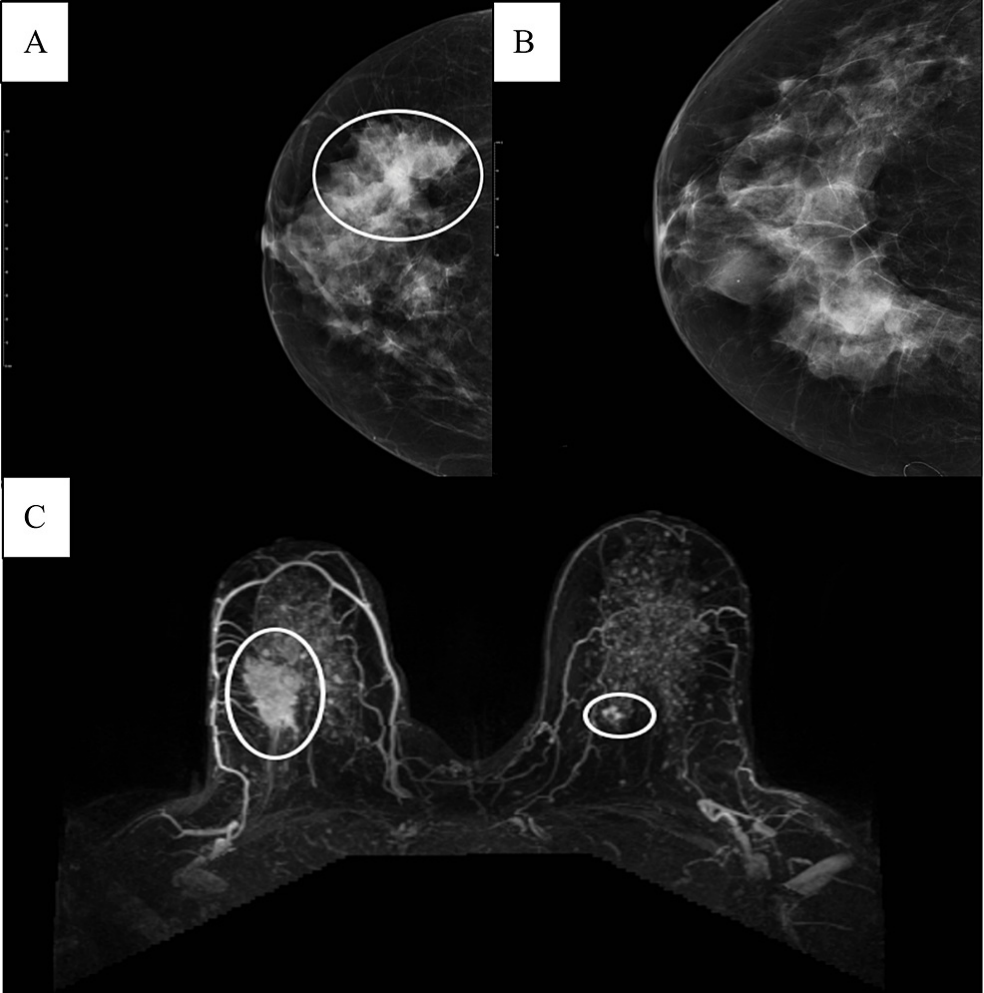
Pre-operative imaging A. Mammography, right breast, cranio-caudal (CC) view, white oval to indicate lesion area B. Mammography, right breast, cranio-caudal (CC) view, no definite findings C. Magnetic resonance imaging (MRI), post-contrast T1, white ovals to indicate lesions bilaterally.
